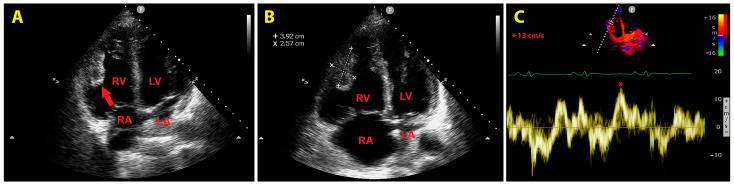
Transthoracic echocardiography. Right-ventricular-focused apical four-chamber view. (A) Large sessile echogenic formation with hyperechoic edges (red arrow), attached to the mid-apical portion of the right ventricular free wall and protruding into the RV cavity. (B) Measurement of the right ventricular mass size. (C) Assessment of the right ventricular mass peak antegrade velocity by pulsed wave tissue Doppler imaging. The right ventricular mass showed a pattern of incoherent motion, totally discordant and independent from the surrounding myocardial tissue. LA, left atrium; LV, left ventricle; RA, right atrium; RV, right ventricle. The symbol * indicates the right ventricular mass peak antegrade velocity.
